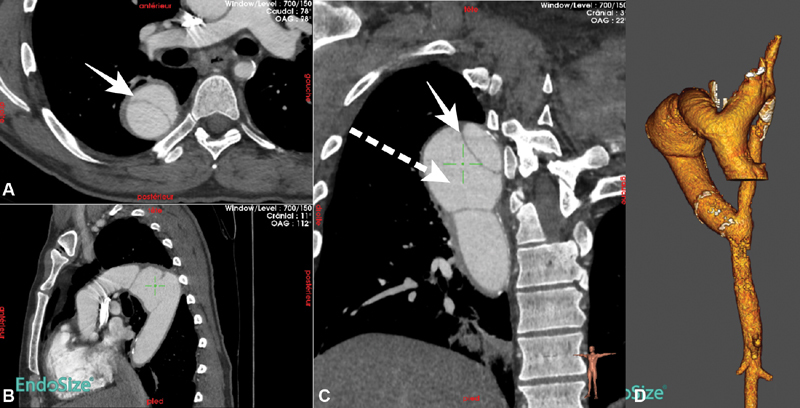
Preoperative computed tomography scan findings with three-dimensional (3D) reconstruction, revealing dilatation of the Dacron graft and a pseudoaneurysm of the end-to-end anastomosis between the two Dacron grafts. (
